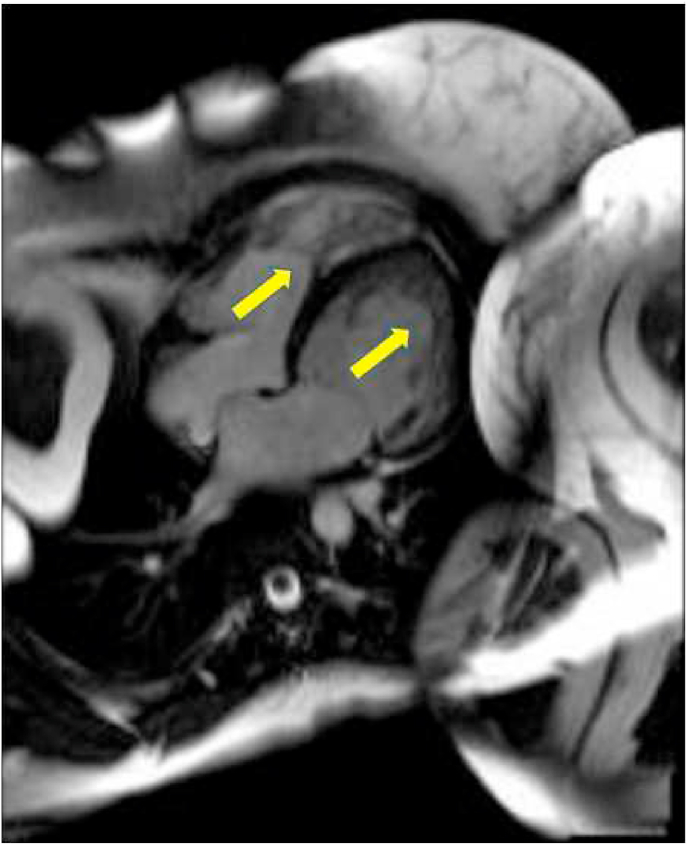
Cardiac magnetic resonance imaging steady-state free precision still-frame 4-chamber view showing left and right ventricle non-compaction (arrows).
